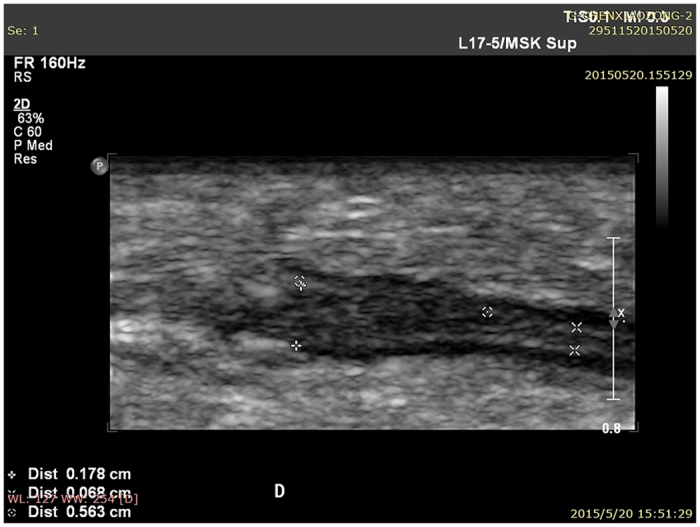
The distal anastomosis features of the regenerated nerve in high-frequency ultrasound.
